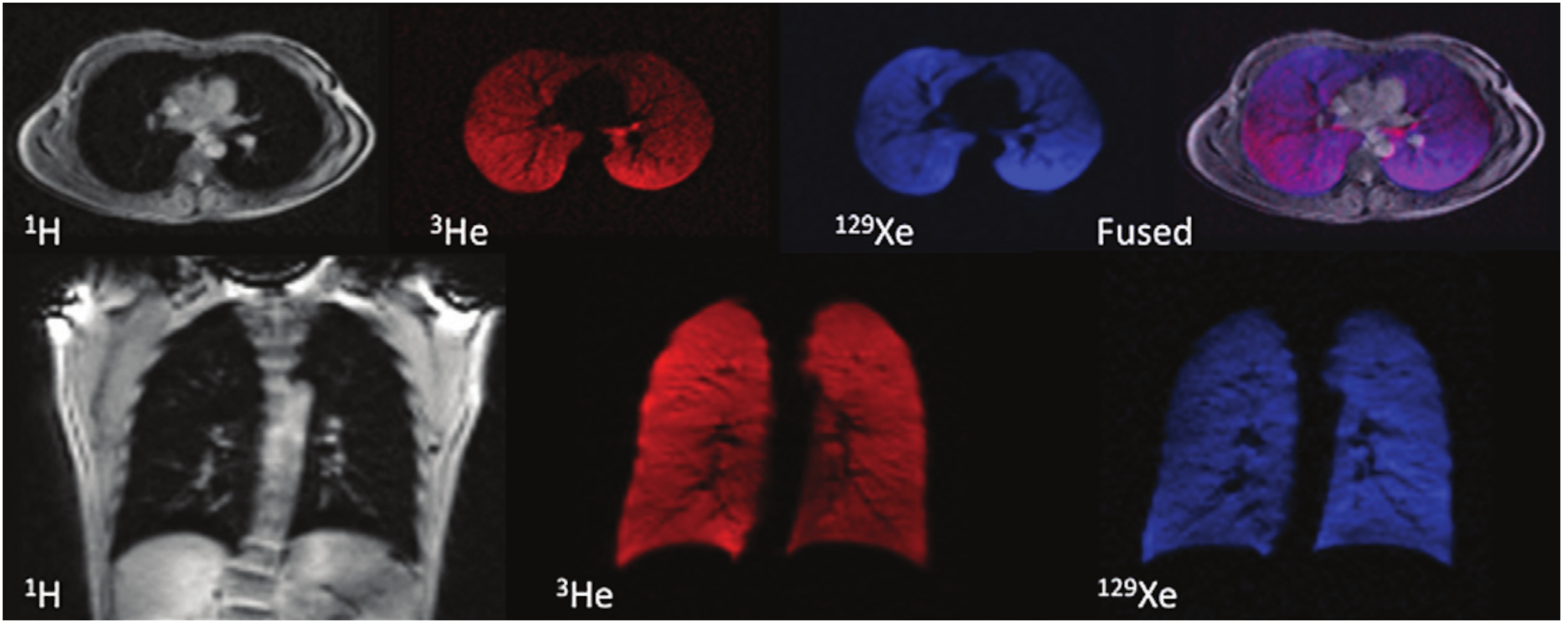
MR images of ^1^H (grey), ^3^He (red) and ^129^Xe (blue) acquired from a healthy volunteer in the same breath‐hold containing 600 mL of ^129^Xe and 300 mL of ^3^He. The anatomic ^1^H images show excellent spatial registration with the ^3^He and ^129^Xe ventilation images, as demonstrated by the overlaid fused image (purple). Figure reproduced with permission from Reference^37^
